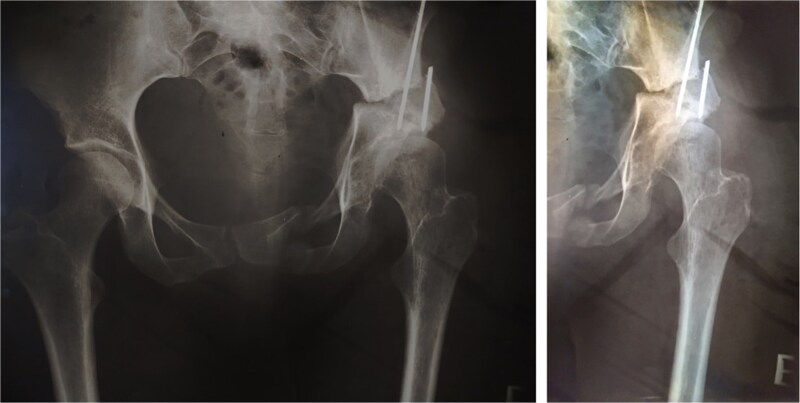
Postoperative anteroposterior radiographs of the pelvis, showing Pol Le Cœeur triple pelvic osteotomy performed on a 35-year-old female patient to treat an acetabular dysplasia in the left hip, at Grade 1 Crowe’s classification.
